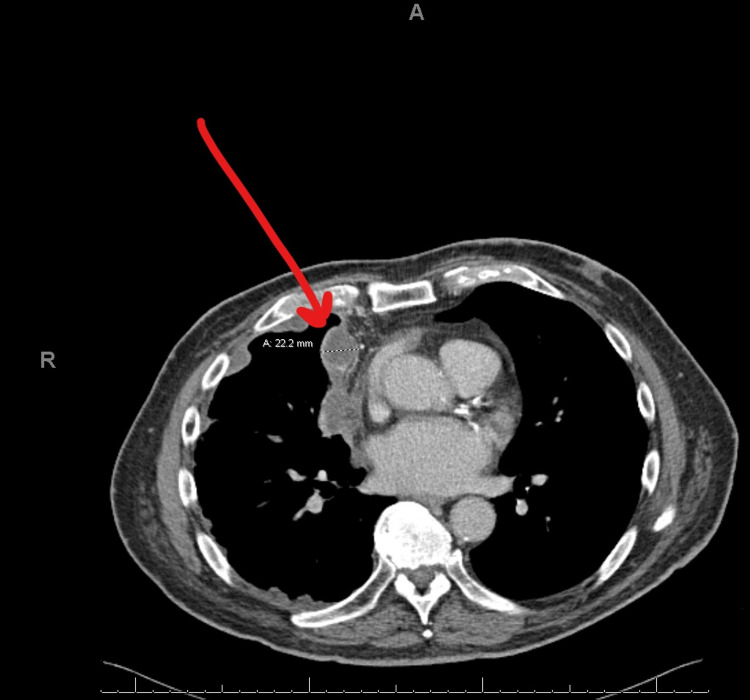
Chest CT examining the progression of the disease with the pleural-based mass medially to the right middle lobe measuring 2.2 cm CT: computed tomography.
